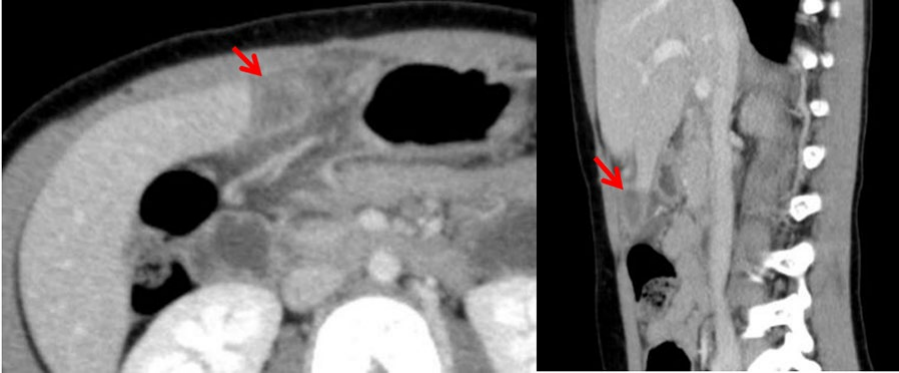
Enhanced computed tomography scan of the abdomen. A low-attenuation mass surrounded by a hyperdense line and disproportionate fat stranding on the right side of the round ligament of the liver
